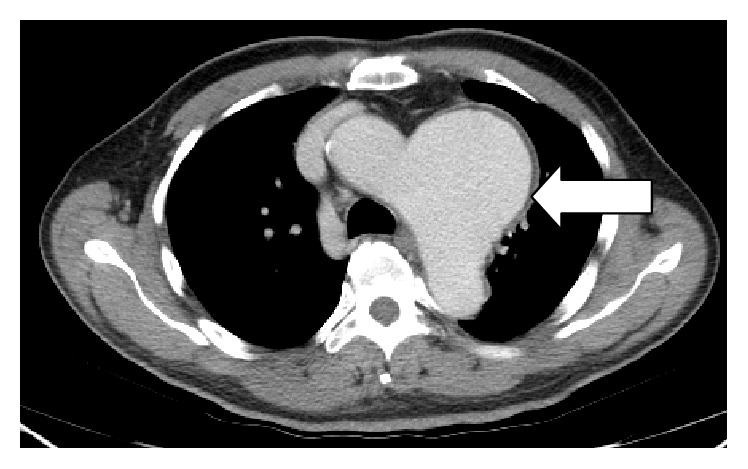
Transverse chest CT scanning, arrow indicated the aortic aneurysm.
